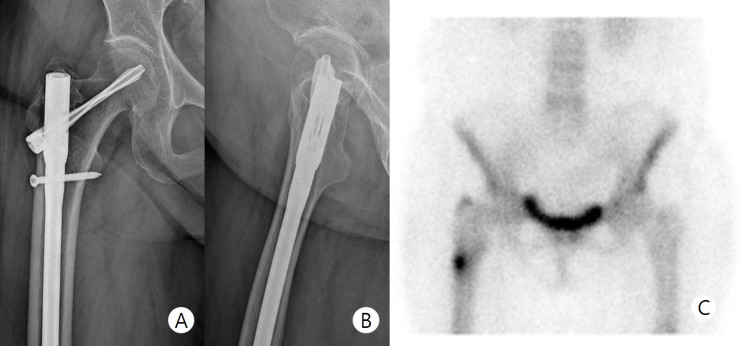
Preoperative imaging - case 1 Preoperative anteroposterior (A) and lateral (B) radiographs show no specific finding. Bone scan (C) shows hot uptake at lateral femoral cortex at the subtrochanteric region
